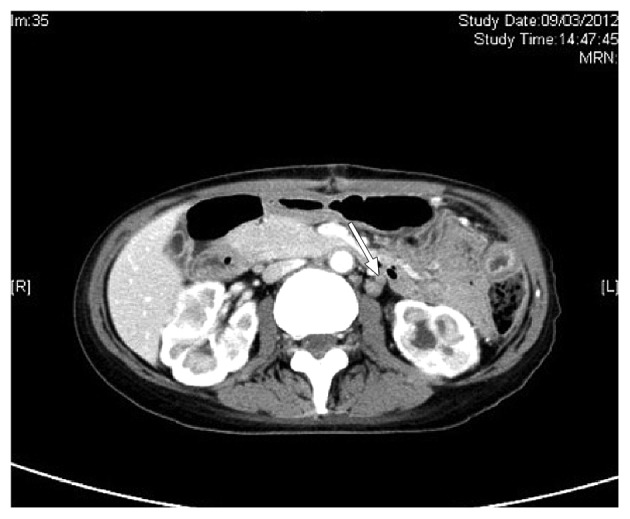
Computed tomography (CT) scan of the abdomen and pelvis. Arrow indicates enlarged paraaortic lymph nodes.
